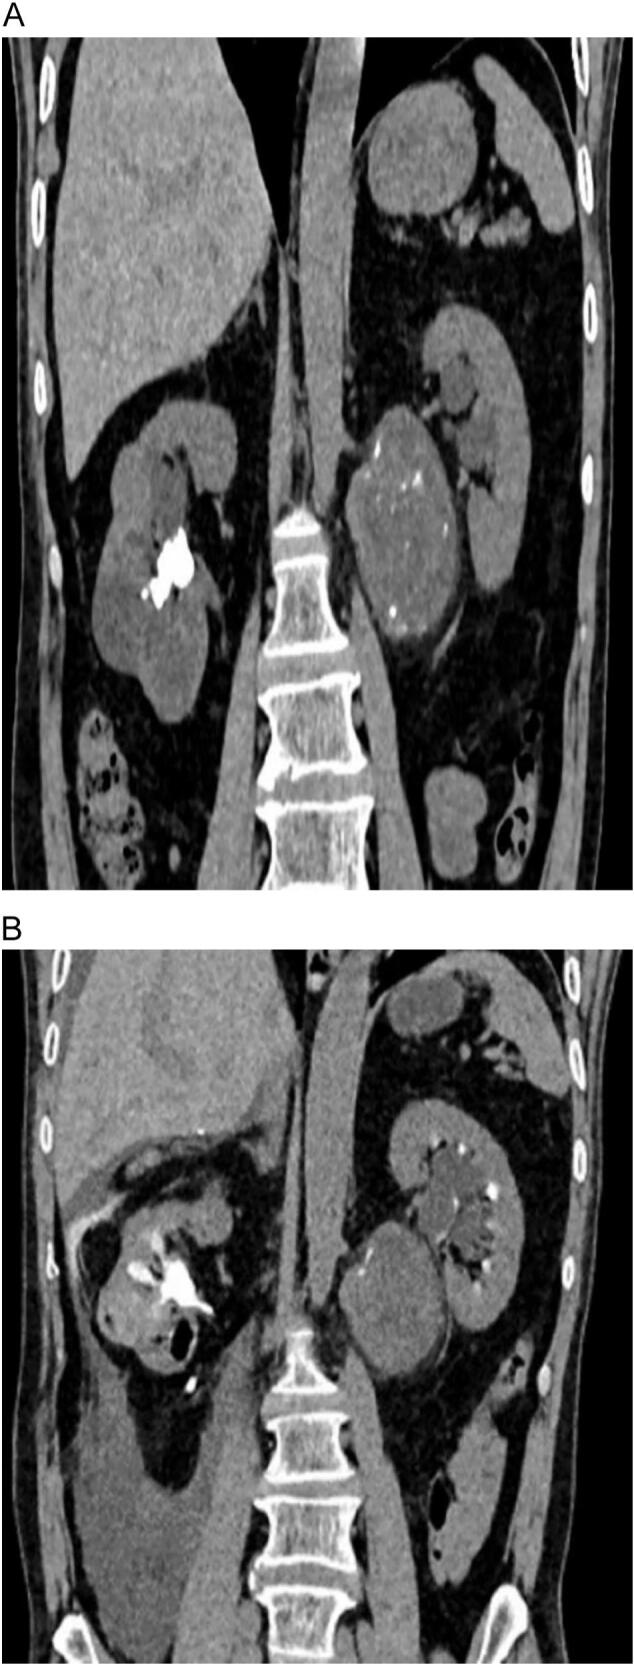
A. CT shows staghorn stone in right kidney and unknown origin mass beside left kidney. B. contrast enhanced CT showed no enhancement.
